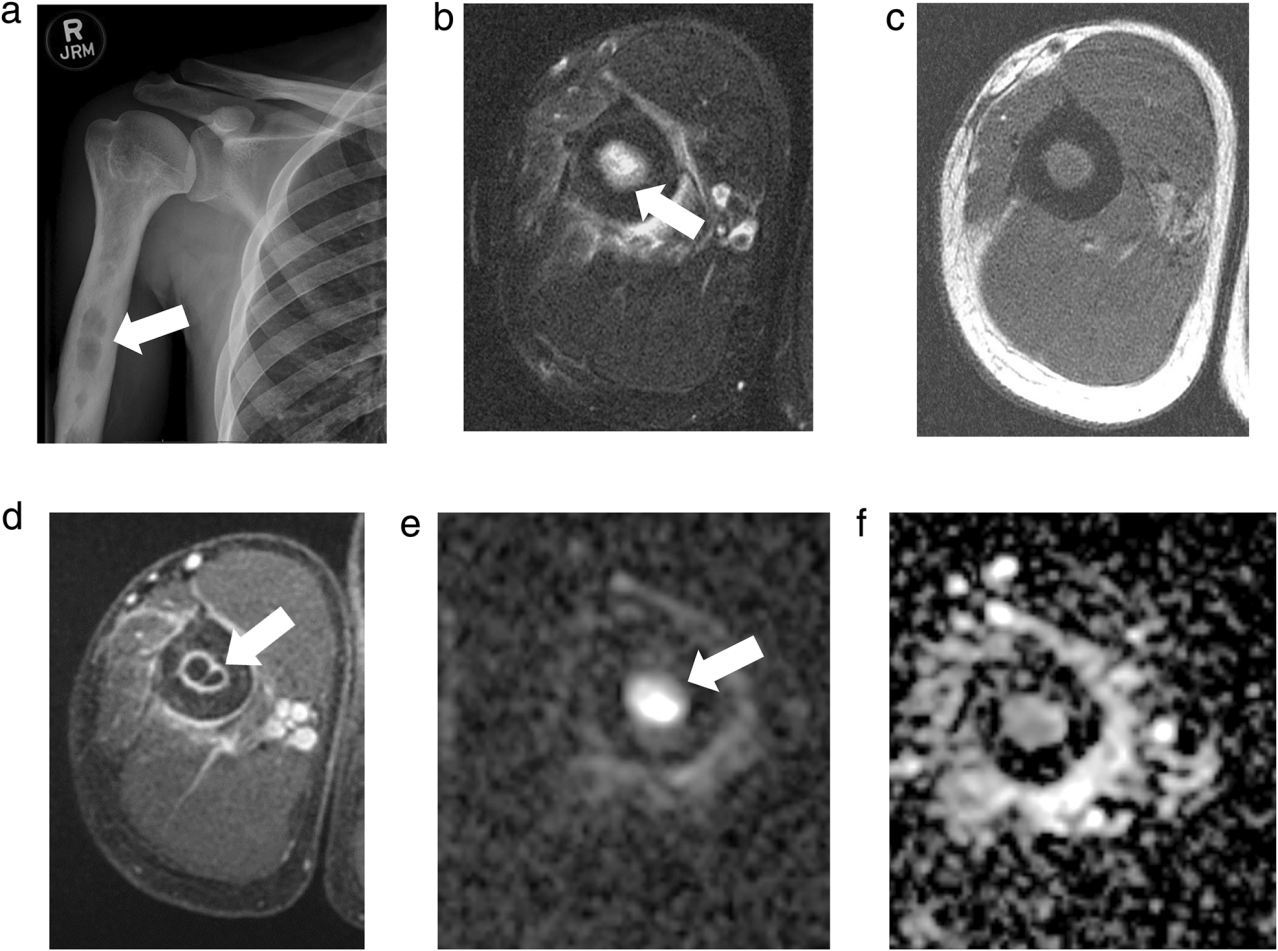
MSKI-RADS Class Va. A 30-year-old man with right arm infection. a Frontal X-ray of the right humerus showing an irregular lytic lesion (arrow), concerning for an intra-osseous abscess. b–d Axial fsT2W (Dixon water map), T1W, and 3D T1W post-contrast images, respectively, of right humerus showing peripherally enhancing intra-osseous abscess (arrows), consistent with subclass Va. e, f Corresponding DWI and ADC map of right humerus showing the associated diffusion restriction
